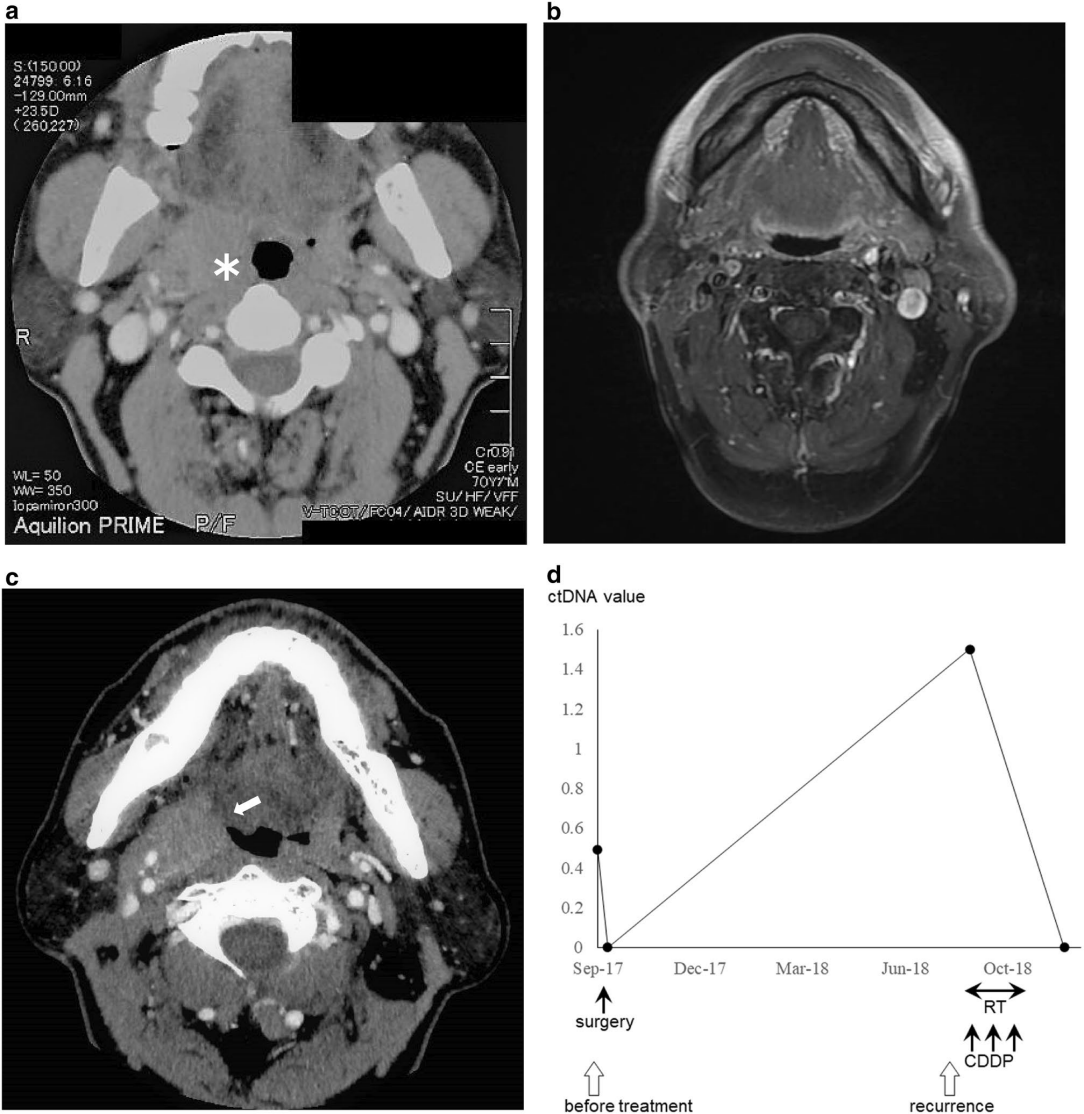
(a) Pretreatment computed tomography (CT) image shows the tumor (asterisk) at the right lateral wall of the oropharynx. (b) Magnetic resonance imaging scan after initial treatment shows complete response. (c) CT image shows the local recurrence of the tumor (arrow) at the right lateral wall of the oropharynx. (d) The pretreatment circulating tumor DNA (ctDNA) was positive and became negative after initial treatment with surgery. ctDNA was positive again at the time of local recurrence and turned negative again after concomitant chemoradiotherapy with CDDP.
